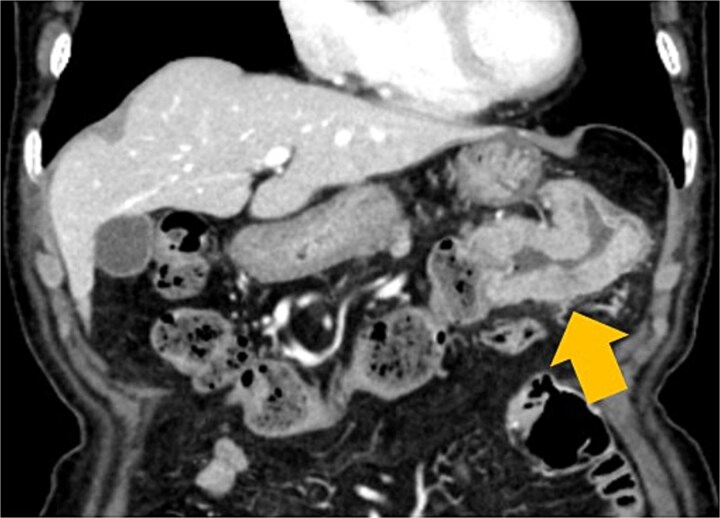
Contrast-enhanced CT findings. Axial view showing a 60-mm mass at the splenic flexure without evidence of distant metastasis.
